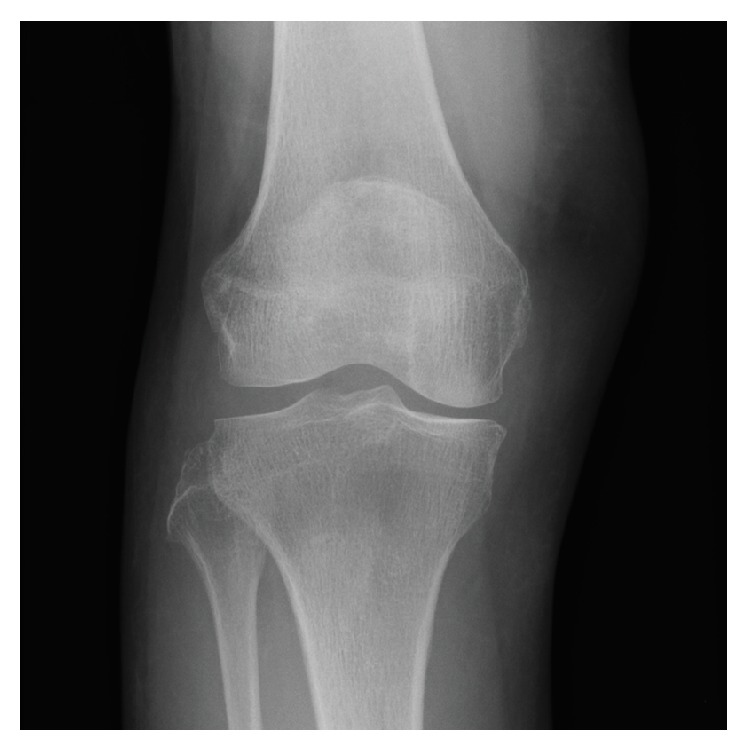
The anteroposterior radiograph of right knee at the onset showing no abnormal finding.
